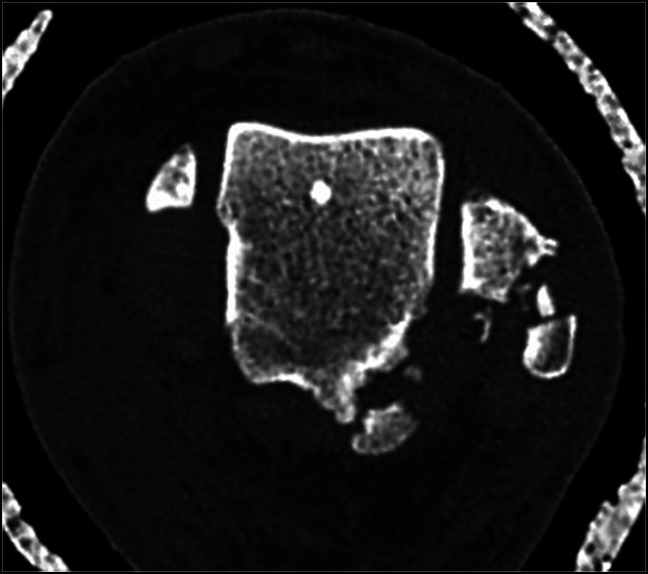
Immediate postreduction axial CT image showing comminuted fracture of the posterolateral talar process and posterolateral fibula.
